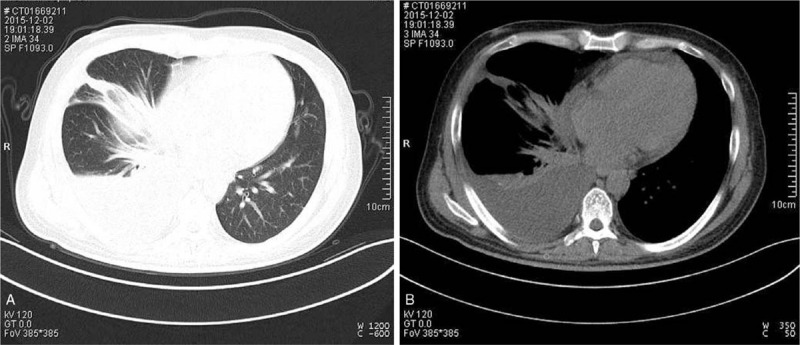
Imaging findings on computed tomography (CT). Chest CT found pleural effusion of the right thoracic cavity and thickening of the right pleura (A-B).
